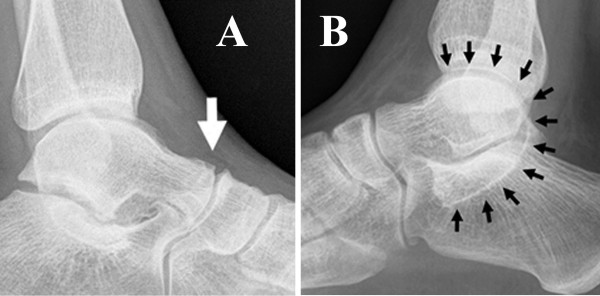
(A) lateral radiograph of a left ankle showing the talar beak (arrow), (B) lateral radiograph of the right ankle obtained of our patient with C sign (arrows) extending from the talar dome through the coalition component of the posterior talocalcaneal joint to the sustentaculum tali.
